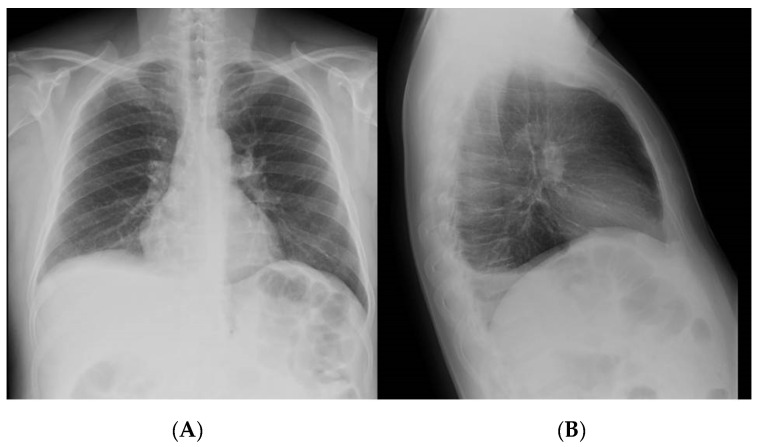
Chest X-ray—right posterior basal pleural effusion as shown in anteroposterior (A) and lateral (B) views.
